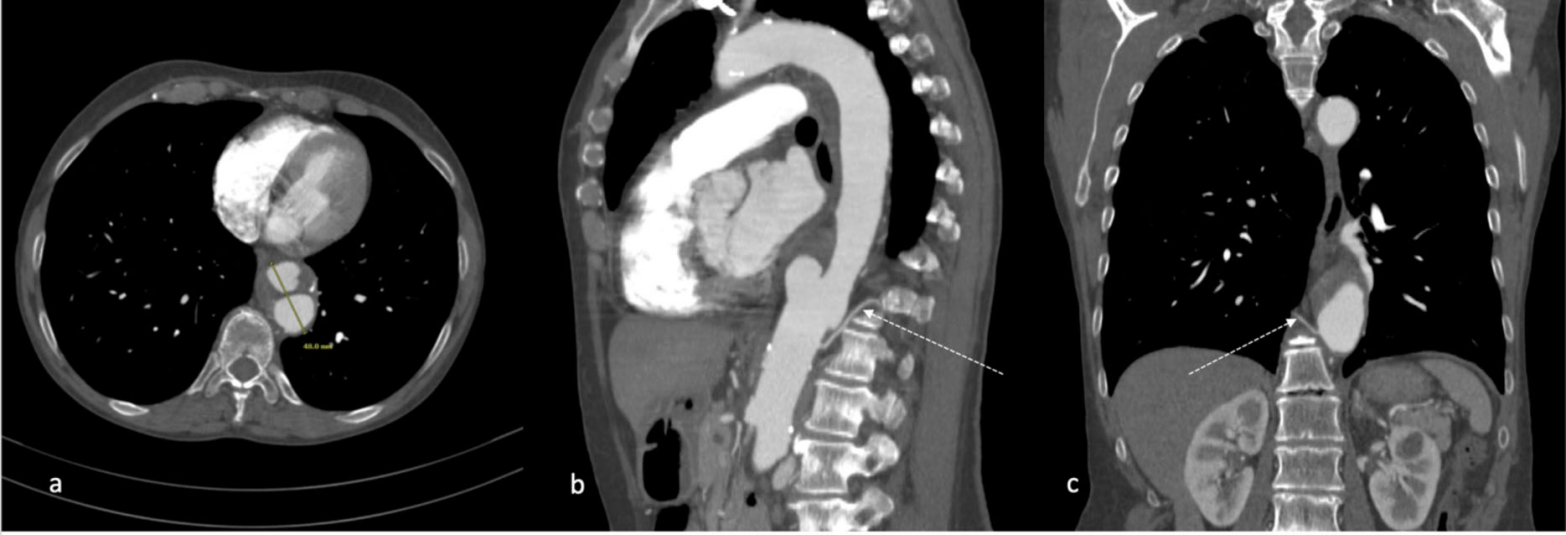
CTA of the aorta. a Axial diameter of the aneurysm. b Sagittal reconstruction with an avid L-1 segmental artery c Coronal projection with a right Th-12 segmental artery. *CTA: computed tomography angiography, L: lumbal, Th: thoracal
